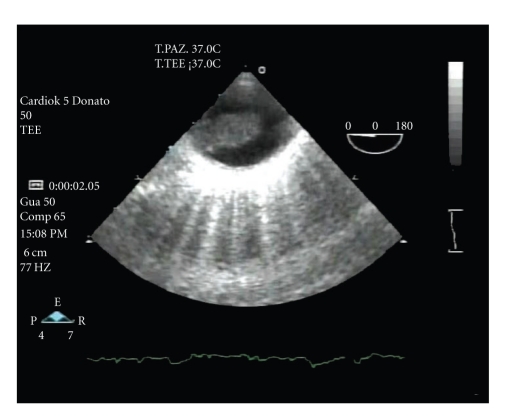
Transesophageal echocardiography documenting the floating mass in descending thoracic aorta.
